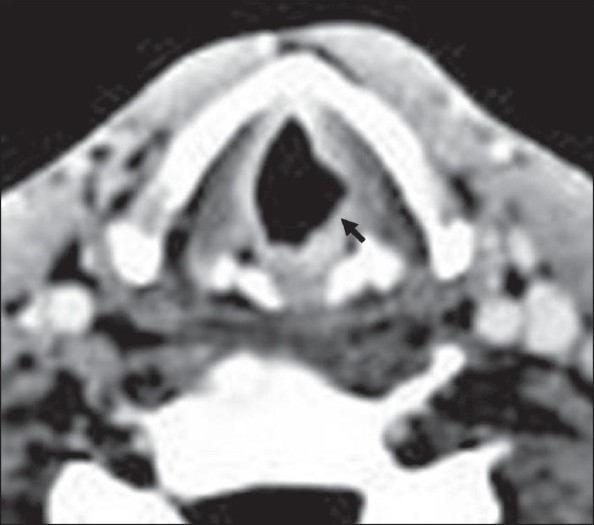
Axial contrast-enhanced CT scan of the larynx shows regular thickening of the left vocal cord (arrow)
